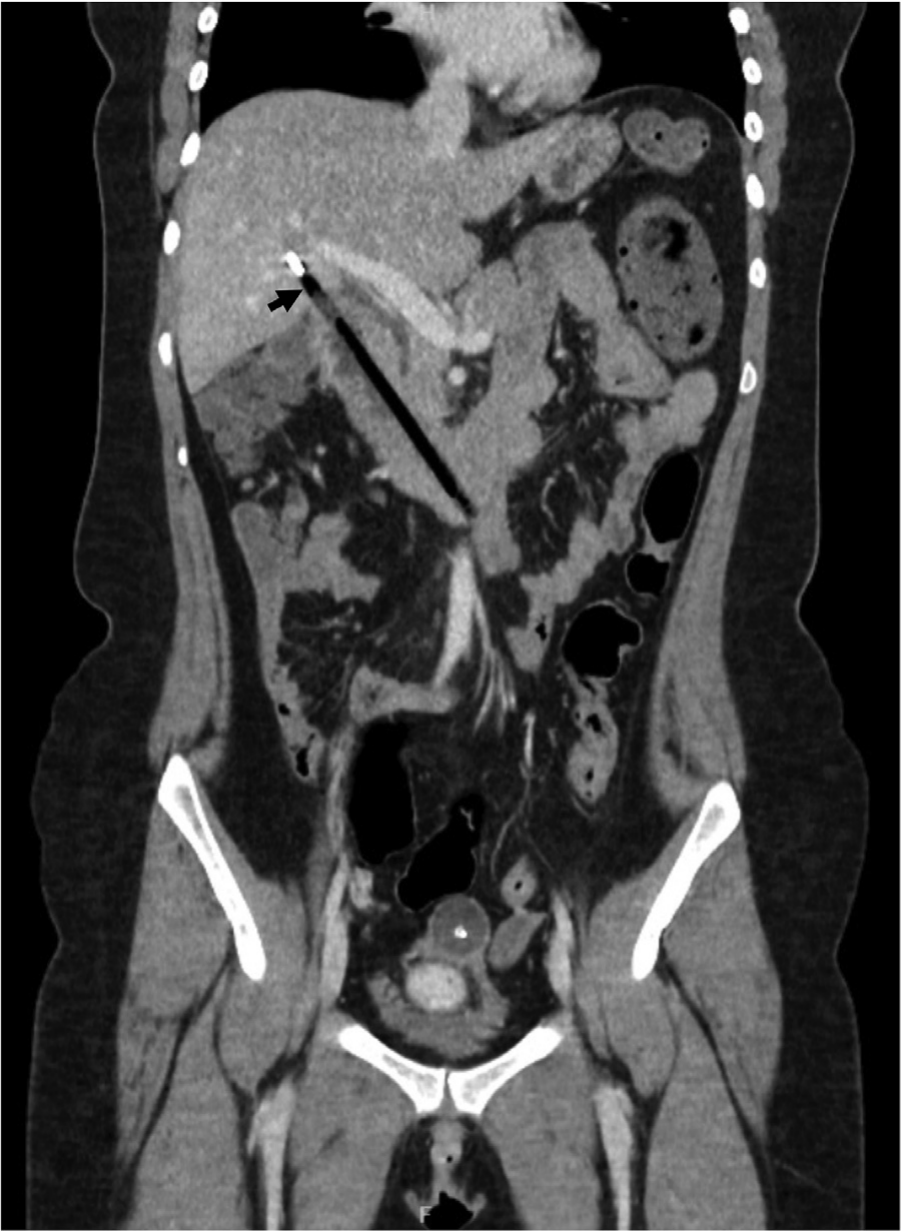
A coronal CT scan view of the abdomen showing fistulization between the duodenum and the hepatic parenchyma with a ballpen in the fistula (black arrow). There was no evidence of free gas or fluid in the peritoneal cavity.
